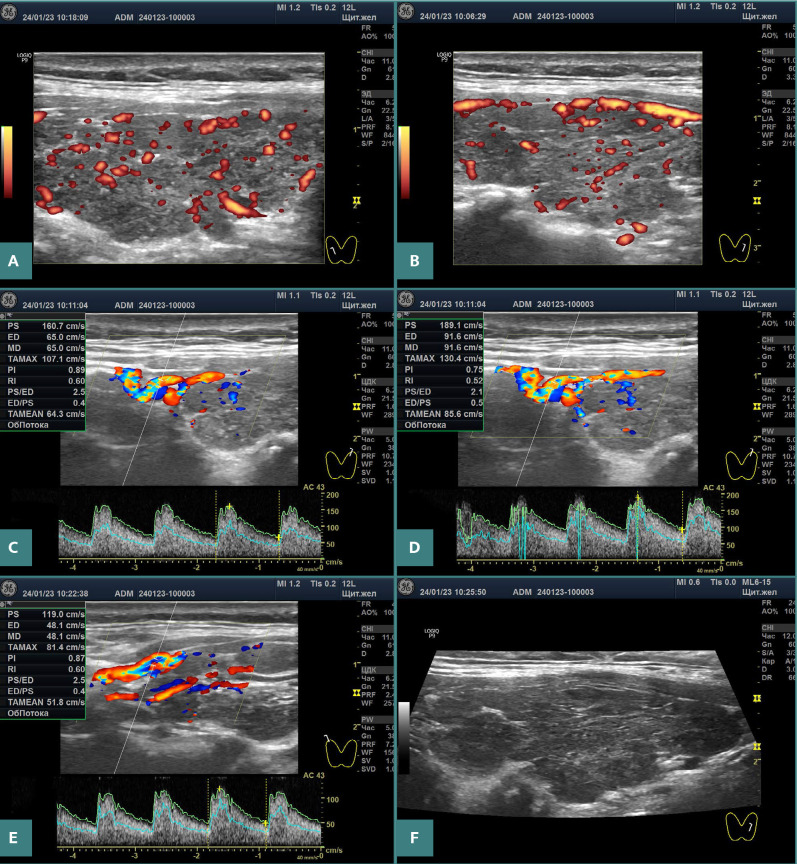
Ultrasound images of the thyroid of patient 3. A,B, Moderate increase in blood flow intensity in two lobes in power Doppler mode at optimal PRF. C, PSV in the first branch of the STAs on the left side at most sites nearly 160 cm/s. D, PSV in the first branch of the STA on the left at one site: 189.1 cm/s. E, PSV in the first branch of the superior thyroid arteries on the right: 119 cm/s. F, Left lobe in grayscale mode.
